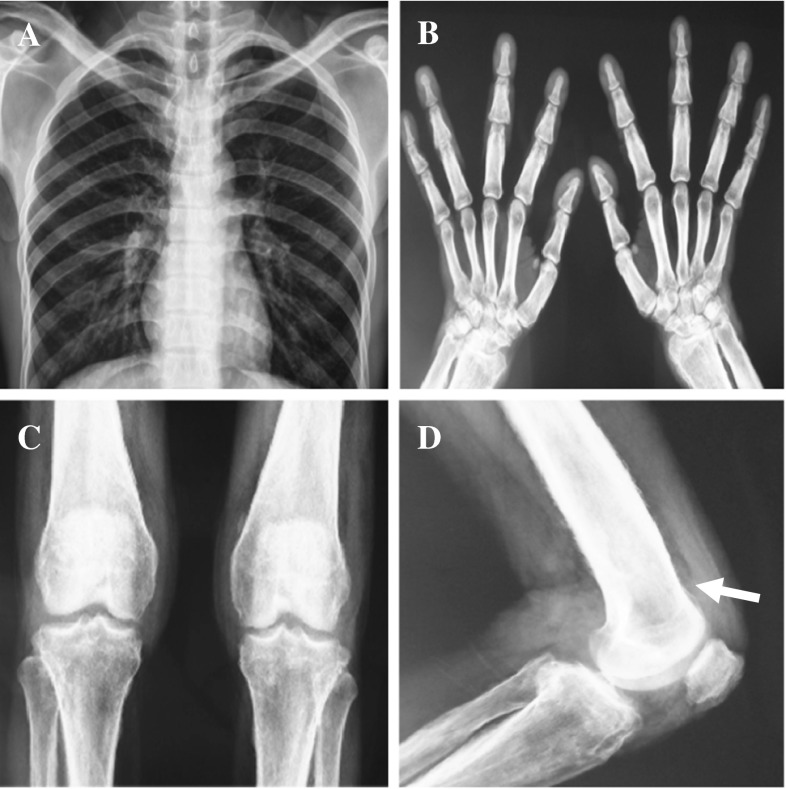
Radiography of the PHO patient with hyperplastic synovium. a Normal X-ray; b both hands showed periostosis; c both legs showed periostitis in the proximal tibia; d pronounced periosteal reaction at the distal end of femur (*arrow*)
